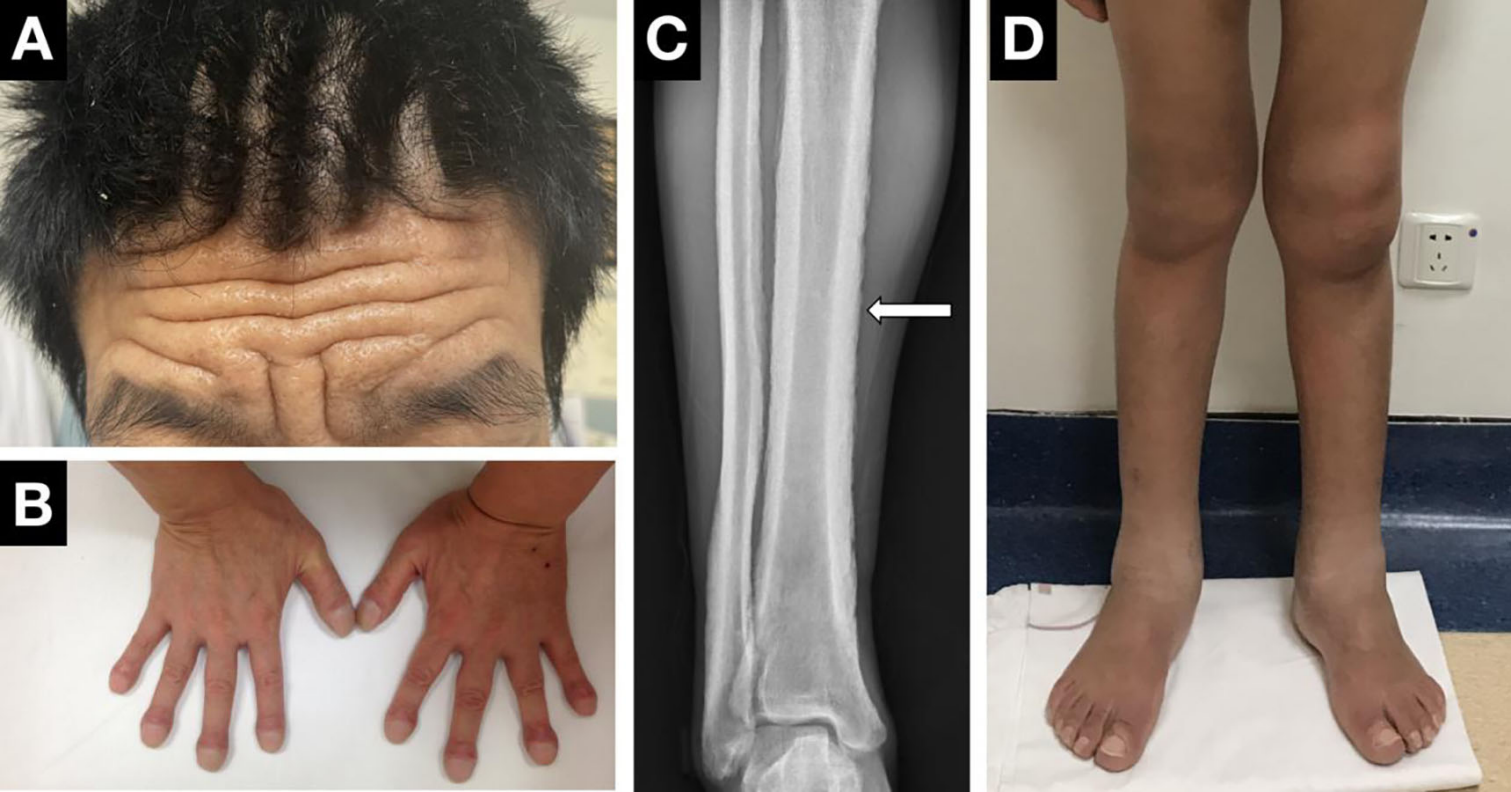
Clinical manifestations of PHO. (A) Pachydermia and cutis verticis gyrate. (B) Digital clubbing. (C) X-ray performance of periostosis. (D) Articular manifestations of the knees and ankle.
